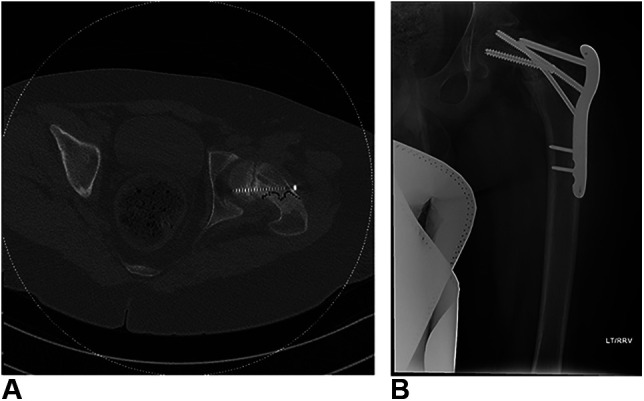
A, Preoperative CT scan demonstrating fracture propagation adjacent to erroneously placed screw tract from index procedure (B) Anterior-posterior radiograph of the revision open reduction and internal fixation
